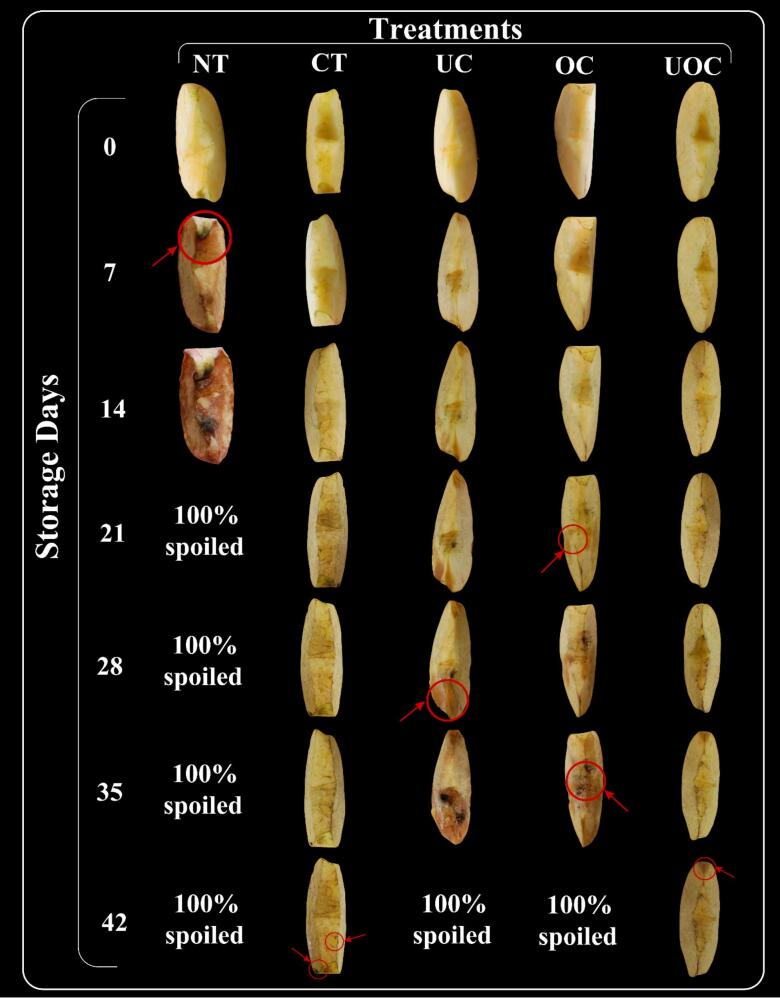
Visual appearance and surface discoloration changes of fresh-cut apples (FCA) samples during storage. Storage intervals included 0, 7, 14, 21, 28, 35, and 42 days. Treatments consisted of NT (untreated control), CT (active edible coating only), UC (ultrasonication followed by active edible coating), OC (ozonation followed by active edible coating), and UOC (combined ultrasonication and ozonation followed by active edible coating). Red indicators highlight representative regions showing browning, discoloration, and visible deterioration during storage.
